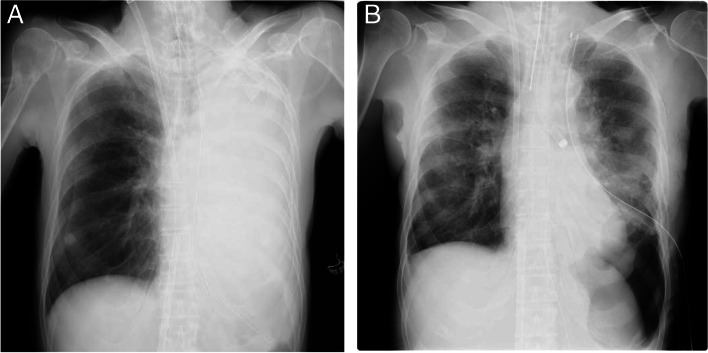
A Chest X-ray taken on day 2. It showed diffuse consolidation of the left lung due to massive hemoptysis, indicating whole lung atelectasis. B Chest X-ray taken after surgery on day 4. It showed good aeration in the resting left upper lung
